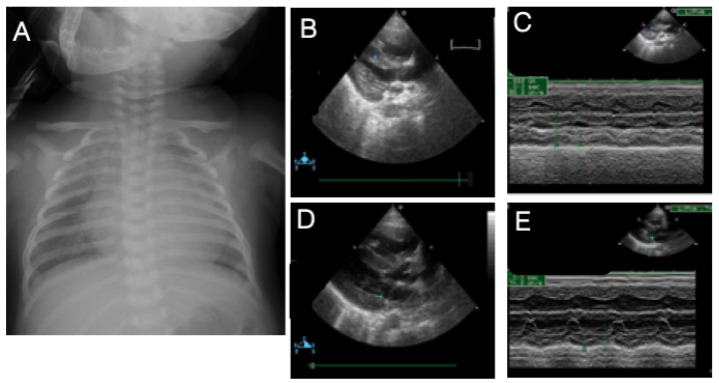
A 3-month-old female infant was diagnosed with pulmonary hypertension with high levels of renin and aldosterone syndrome. Chest radiography showed a mild ground glass appearance and cardiomegaly with a cardiothoracic ratio of 0.75 (A). Long-axis echocardiographic images (two-dimensional (B) and M-mode (C)) demonstrated a hypertrophic right ventricle, which was much improved 6 months after candesartan (angiotensin receptor blocker) and captopril (angiotensin-converting enzyme inhibitor) were given (D,E).
